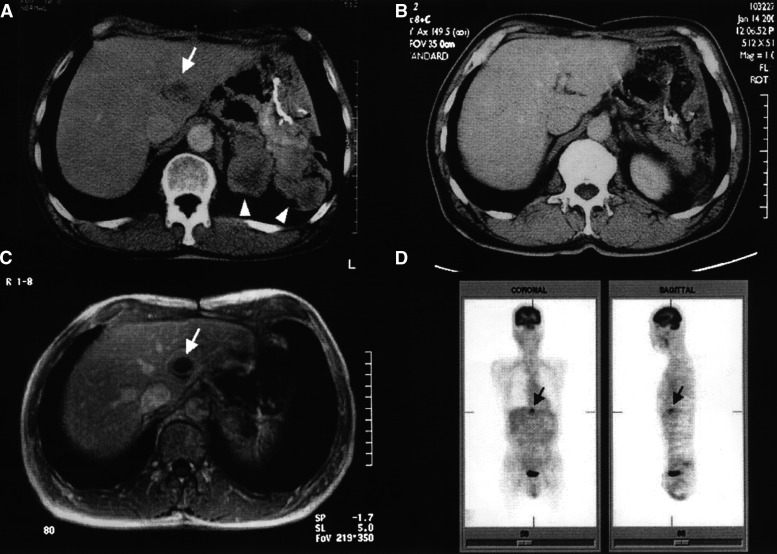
Response to imatinib treatment. Computed tomographic examination before imatinib treatment: a solitary liver metastasis (arrow) and multiple peritoneal metastases (arrowheads) (A), CT scan after 6 months of imatinib treatment showing a partial response (B), gadolinium-enhanced T1-weighted MRI after 9 months of imatinib treatment showing disease progression only in the liver metastatic deposit (arrow) (C), PET scan after 10 months of imatinib treatment. The increased uptake of the tracer [^18^F]FDG was seen only in the liver metastatic deposit (arrow) (D).
